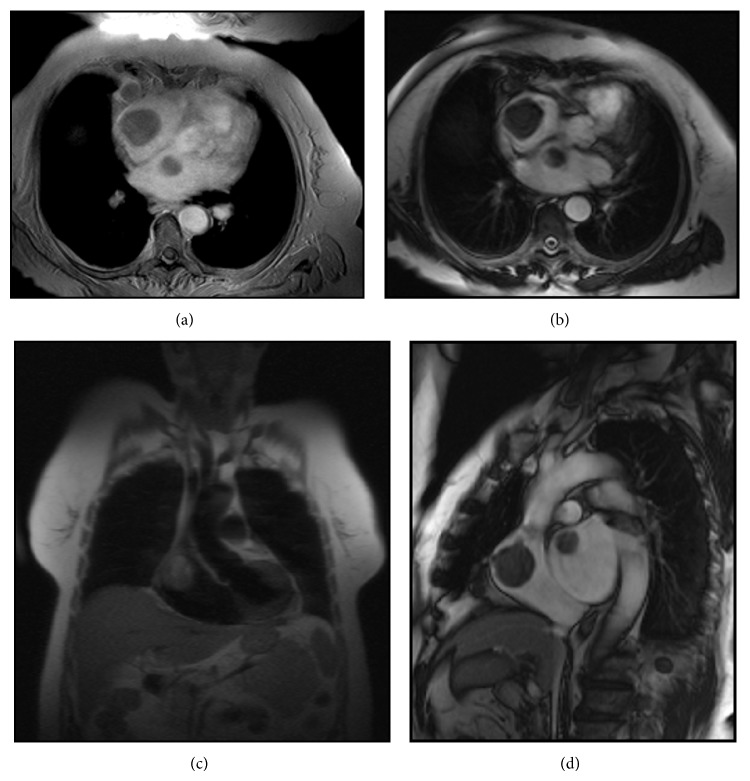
Cardiac MRI demonstrates two masses with biatrial cardiac metastases of malignant melanomas in uterine cervix: one is 37 mm and the other is 20 mm in right and left atriums.
